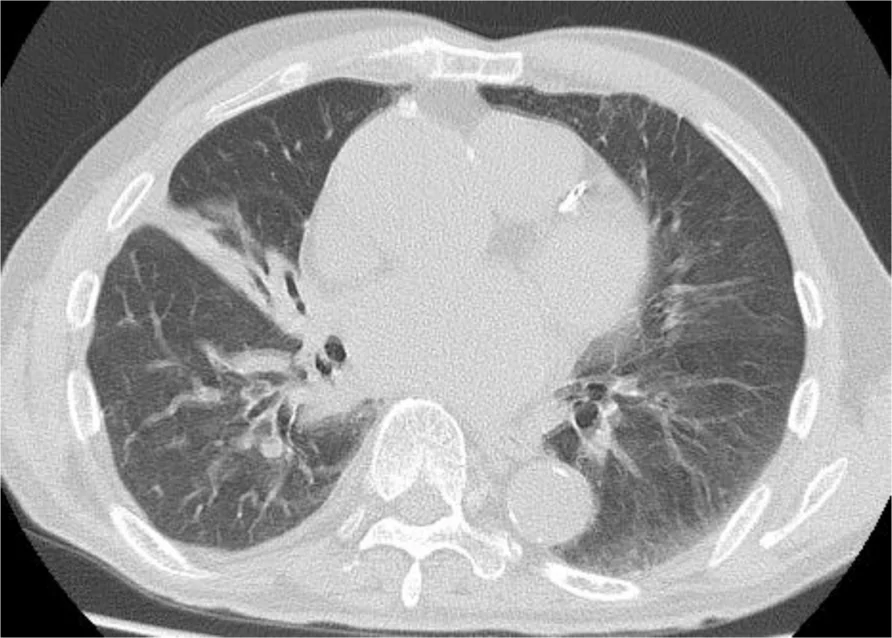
Chest computed tomography obtained 8 months after surgery showing resolution of right middle lobe atelectasis
